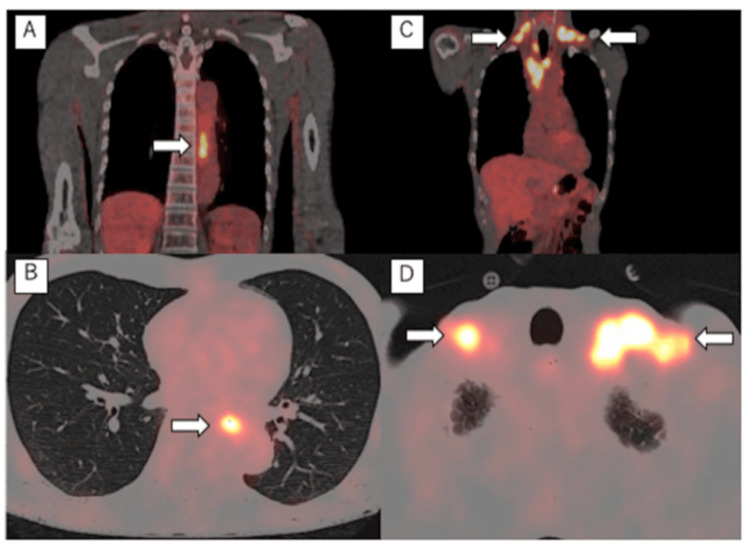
FDG-PET FDG-PET showing an abnormal uptake of FDG in the main tumor (A, B) (maximum standardized uptake value of 8) and lymph nodes (C, D) (maximum standardized uptake value of 10) (white arrows) FDG-PET: 18F-fluorodeoxyglucose positron emission tomography
